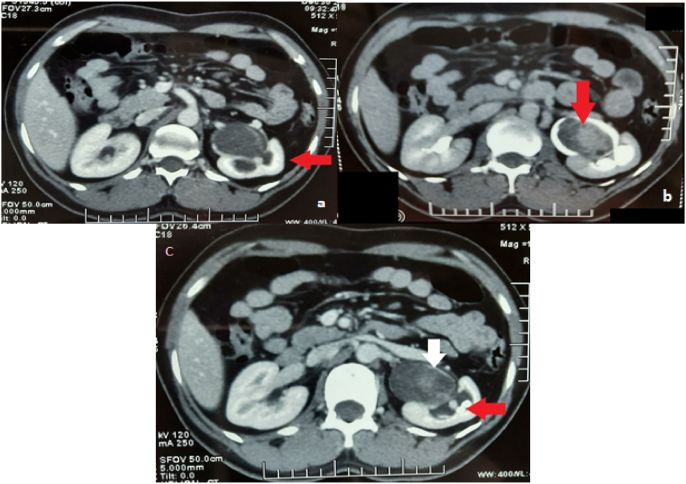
a-c Axial view of CT scan abdomen showing mild to moderate hydronephrosis (red arrow) secondary to pelvic mass (a). Axial view CT scan abdomen showing mass (red arrow) in the pelvis with peripheral enhancement (b). Axial view of CT scan abdomen showing obstruction secondary to pelvic mass (white arrow) and multiple calculi (red arrow) (c). (For interpretation of the references to colour in this figure legend, the reader is referred to the Web version of this article.)
